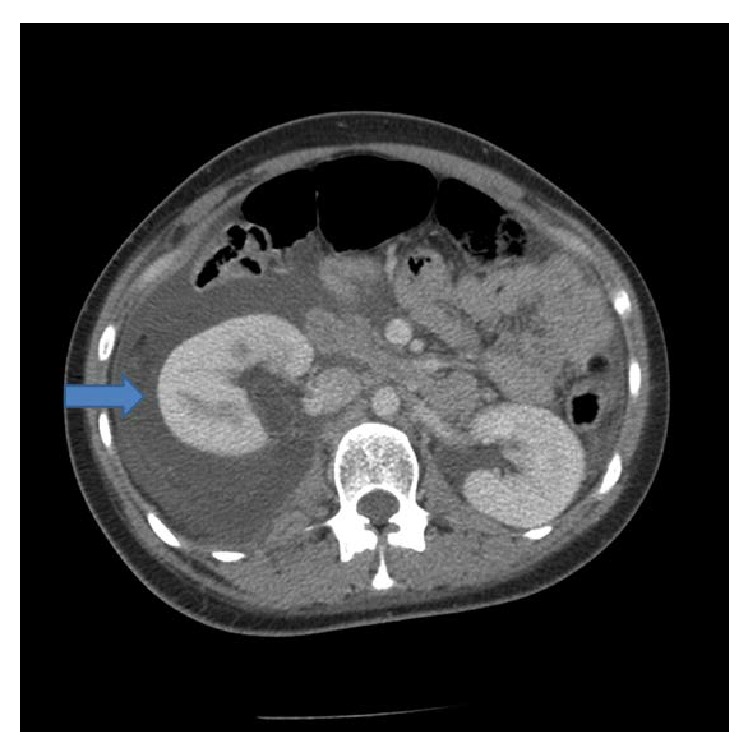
CT scan of abdomen on admission. Blue arrow indicates presence of urinoma.
